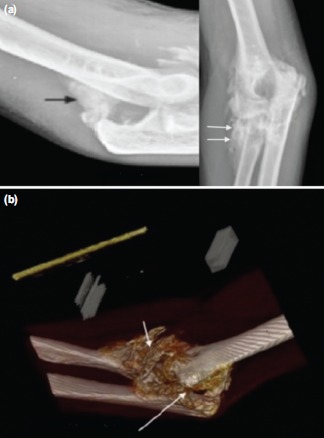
(a) Antero-posterior and lateral radiographs showing HO Hastings IIIC bridging the humero-ulnar (arrows) and radio-ulnar joints (arrows), (b) 3D CT of case shown in Figure 2.
